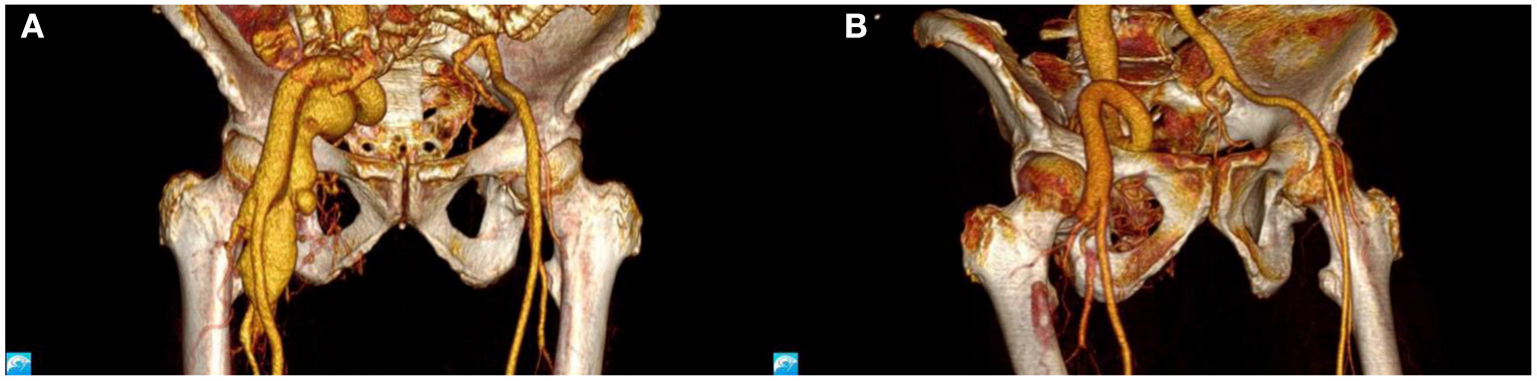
CT angiography, 3D reconstruction: (A) before and (B) after the operation.
